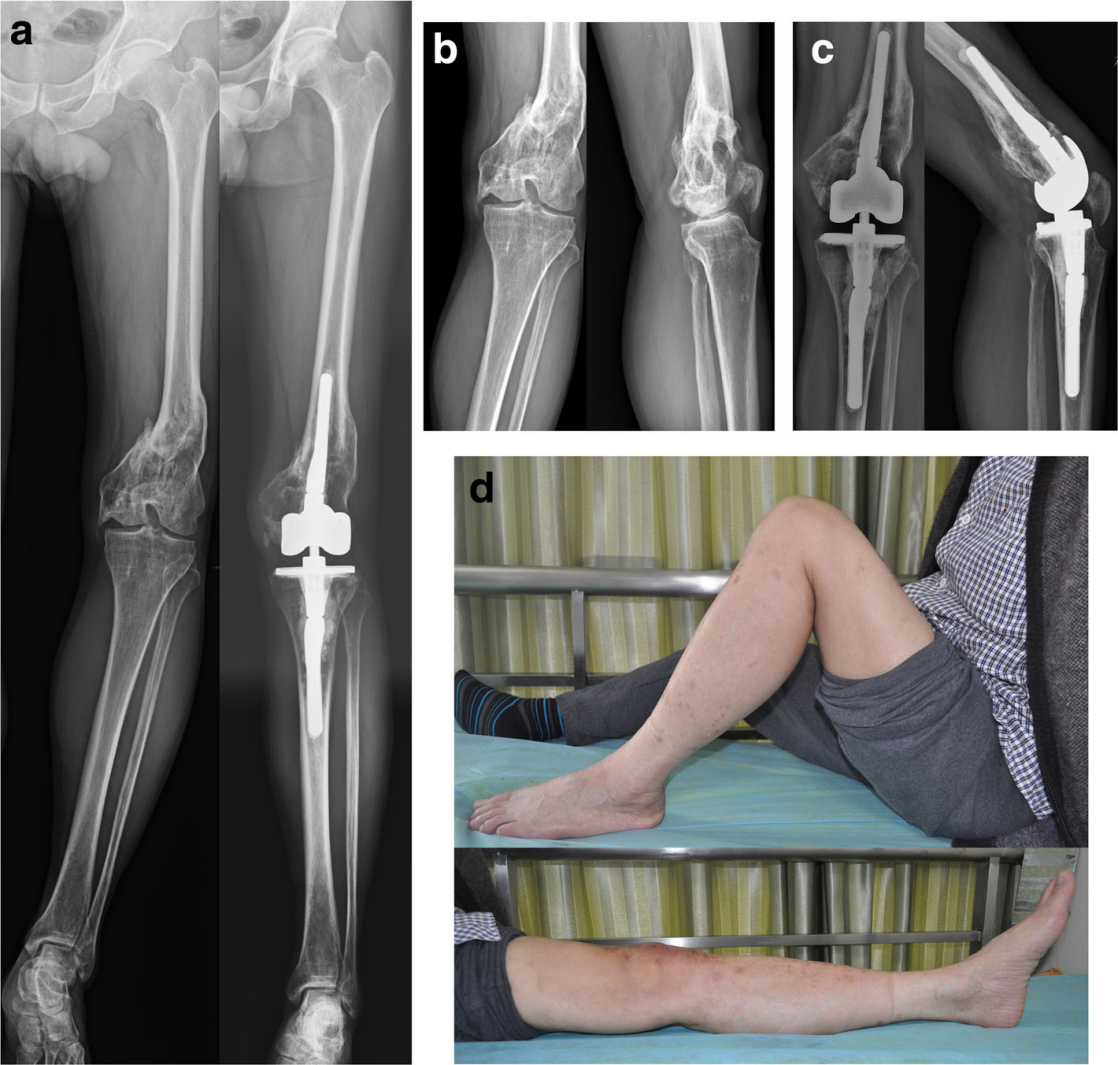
CCK prosthesis in a 58-year-old male patient with the mal-united distal femur and post-traumatic arthritis of the left knee. The patient was having knee stiffness and patella baja for 20 years due to post-traumatic scar. a Comparison of pre-operative and post-operative long leg radiographs in anteroposterior (AP) view. b, c Pre-operative and post-operative radiographs respectively. d Clinical photographs showing a post-operative range of motion (ROM)
